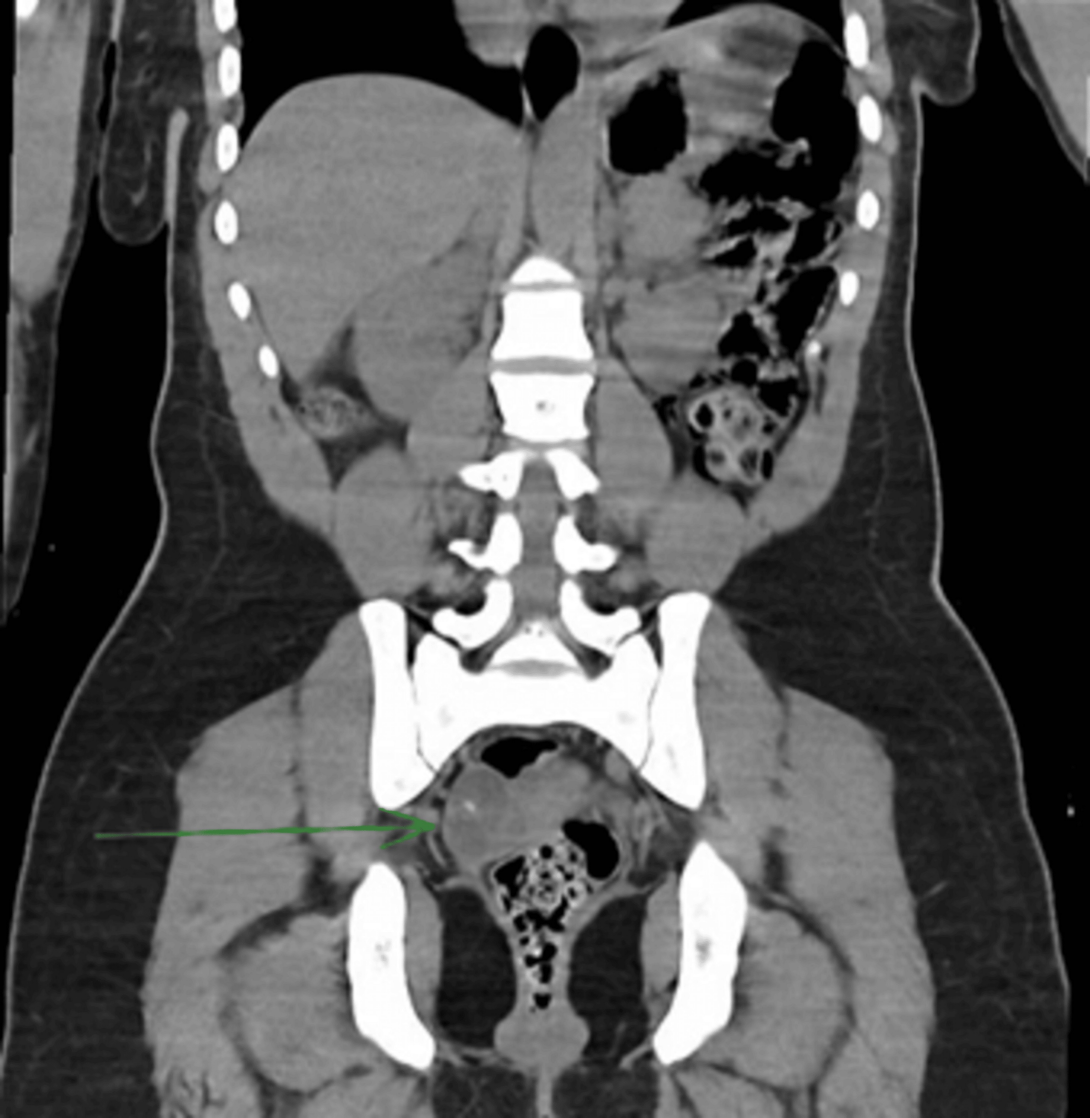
Coronal CT scan showing a 6.1 cm right ovarian teratoma (green arrow). CT: Computed tomography
